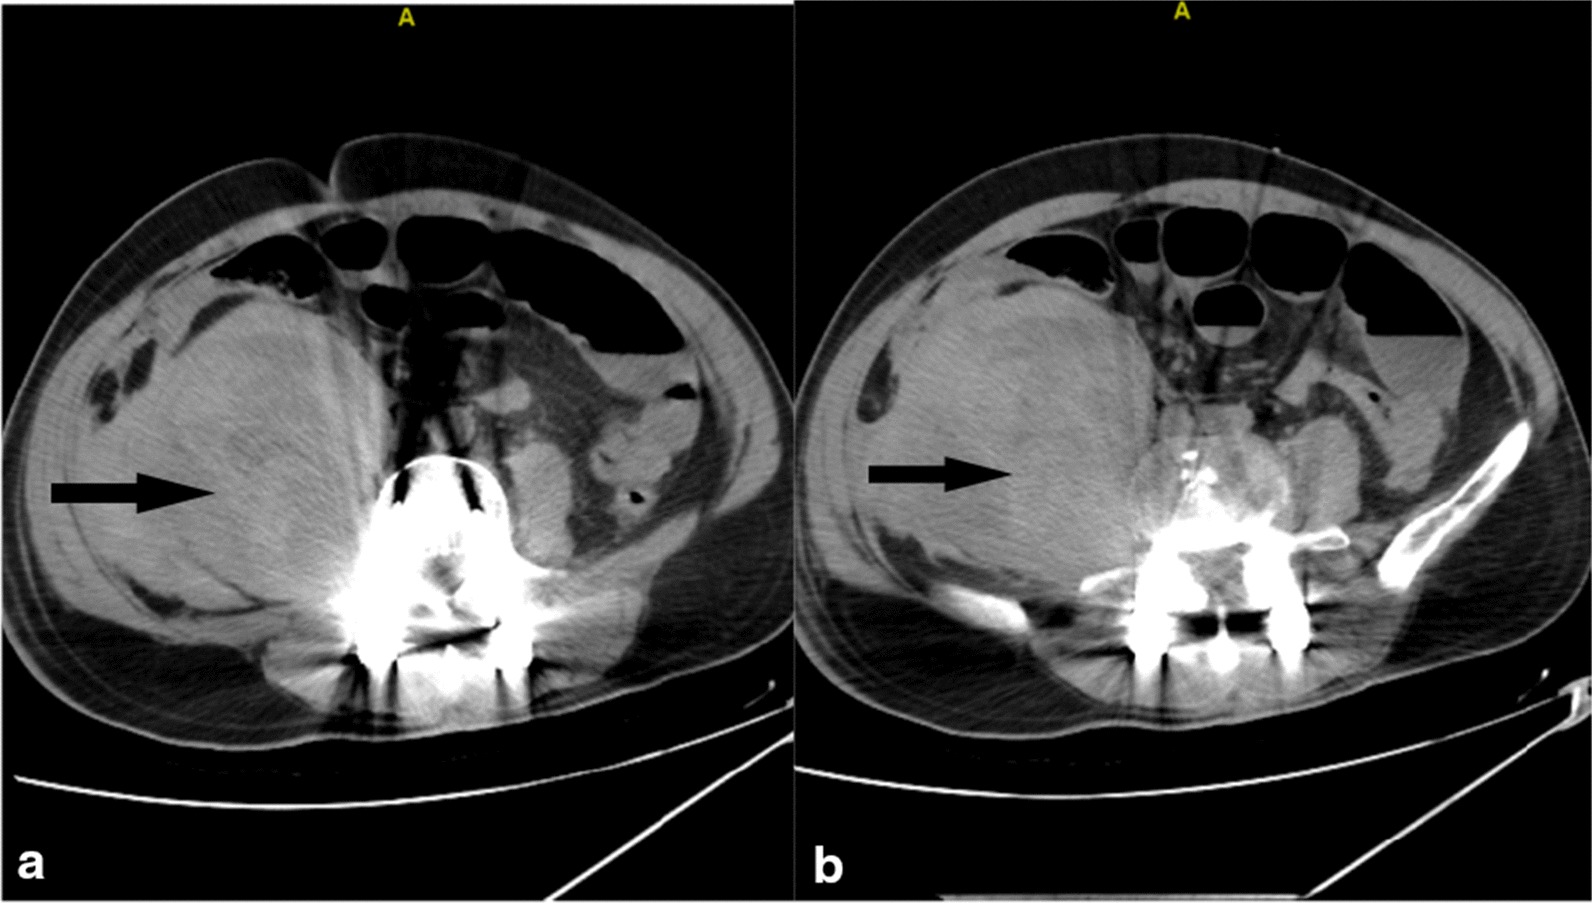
Abdominal CT scan 20 days post- surgery showing prompt psoas hematoma
